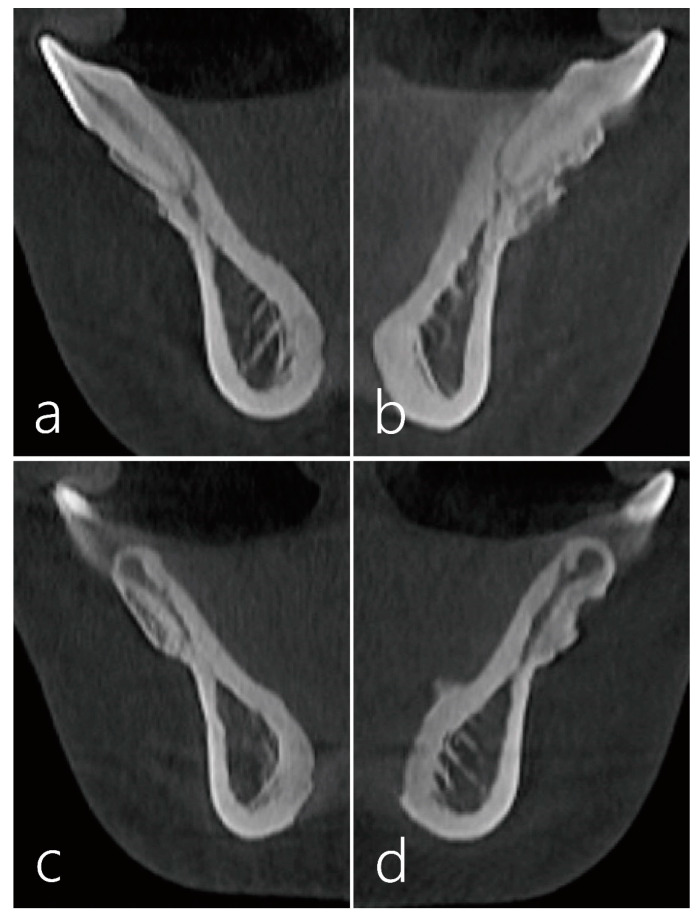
(a,b) The cross-sectional CBCT scans 5 years after the surgery showed well maintained labial bone plate at the midfacial side of both mandibular central incisors. (c) On the cross-sectional CBCT scan at the interdental site between the right lower central and lateral incisors, hard tissue phenotype modification was observed on the labial side. (d) On the cross-sectional CBCT scan at the interdental site between the left lower central and lateral incisors, partial depression was present but hard tissue phenotype was improved overall.
